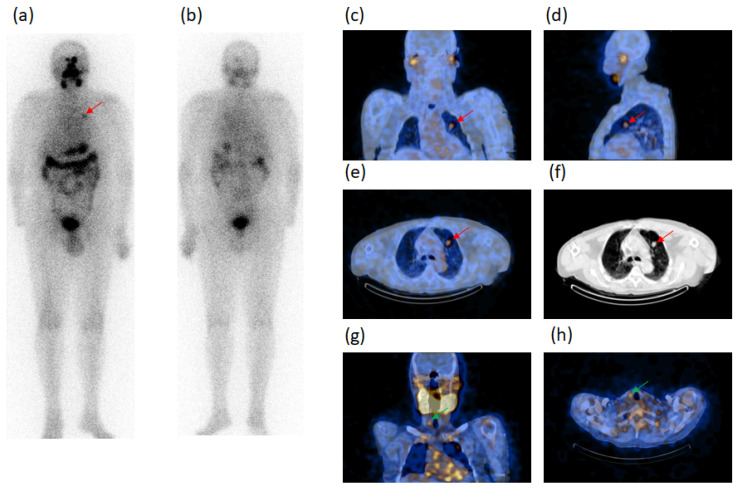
A 73-y-old male patient (case n. 8, Table 3), thyroidectomized for thyroid papillary carcinoma, follicular variant, tumor diameter 25 mm, at the first radioiodine ablation. ^131^I-WBS after a therapeutic dose, in both anterior (a) and posterior (b) views, showed one radioiodine-avid focus in left hemithorax suspected of metastasis (red arrow). Fused SPECT/CT images in coronal (c), sagittal (d) and transaxial (e) slides confirmed the focus (red arrows) specifying the anatomic site (anterior segment of the superior lobe) and characterizing it as lung metastasis, as also confirmed at CT (f). In addition, fused SPECT/CT images in coronal (g) and transaxial (h) slides evidenced another radioiodine-avid focus (green arrows) in the neck corresponding to a right paratracheal lymph node occult at WBS and classified as metastasis. Thyroglobulin levels in hypothyroidism condition: 1362 ng/mL. AbTg: Absent.
